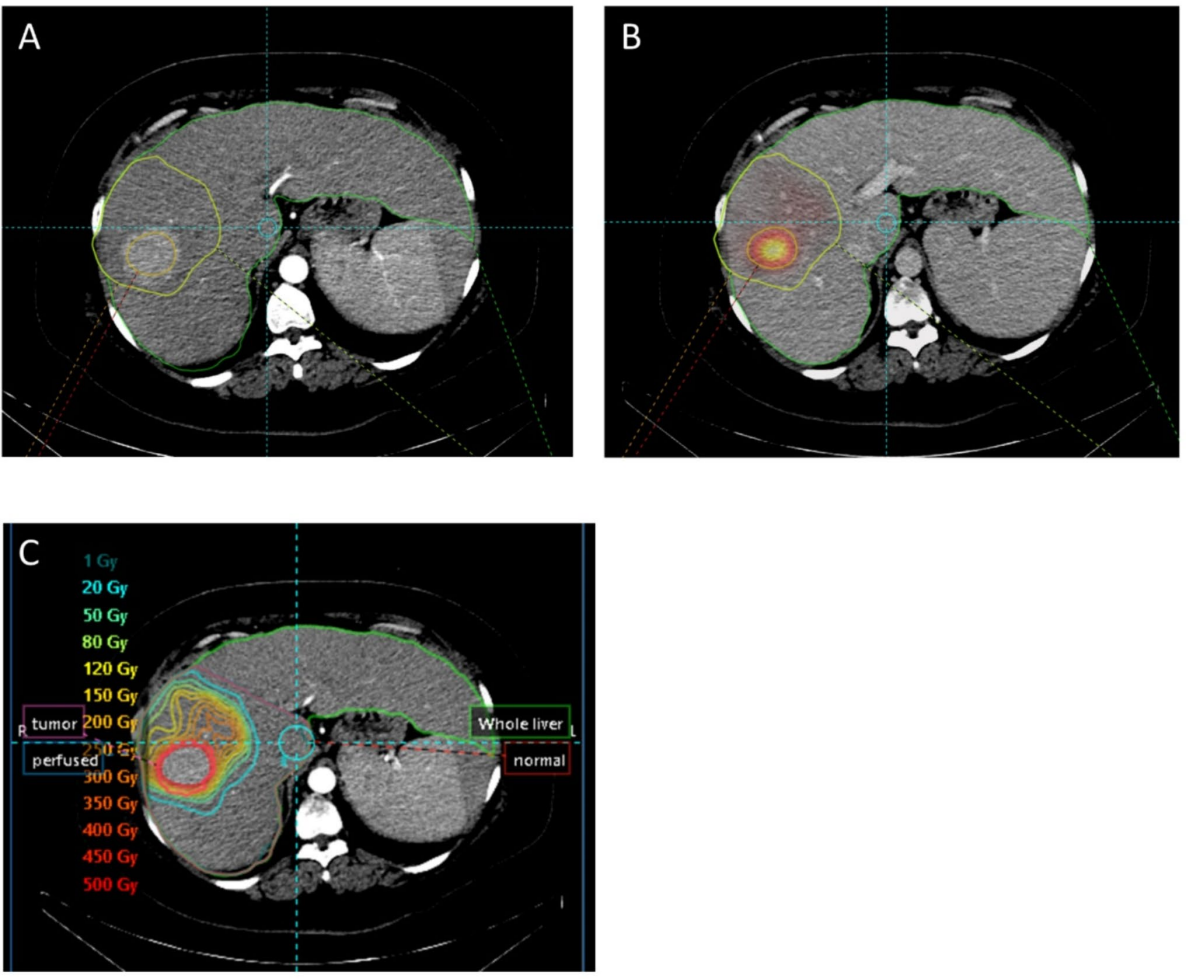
In most cases, after proper co-registration, the hypervascular tumor segmented on CT/MRI (A) closely matches the high focal accumulation of [^99m^Tc]TcMAA on SPECT/CT (B), resulting in high tumor-absorbed doses and sharp dose drops in surrounding liver tissue (C). Both segmentation methods will be accurate
